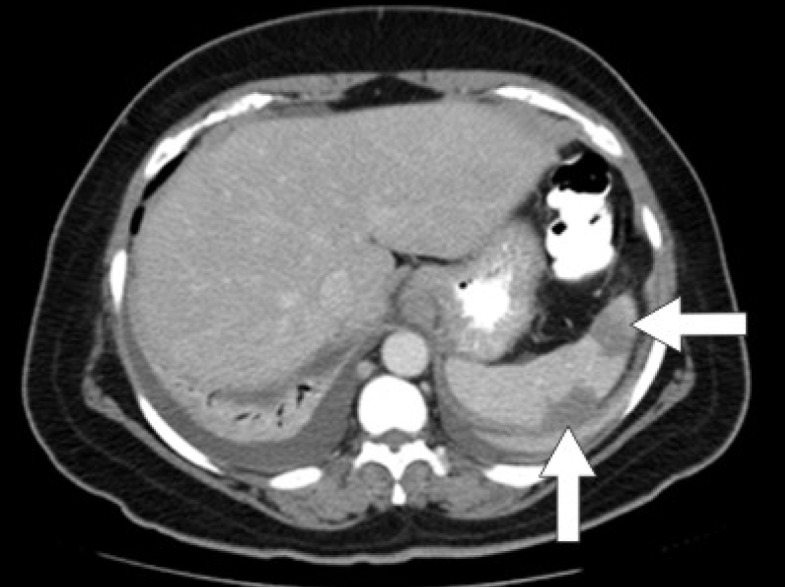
CT scan of the abdomen showing large splenic wedge-shaped hypodensities consistent with splenic infarcts.
